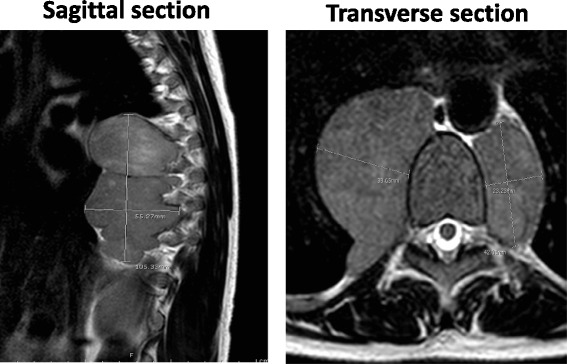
MRI study of the paraspinal masses. Representative images of the paraspinal masses were shown
